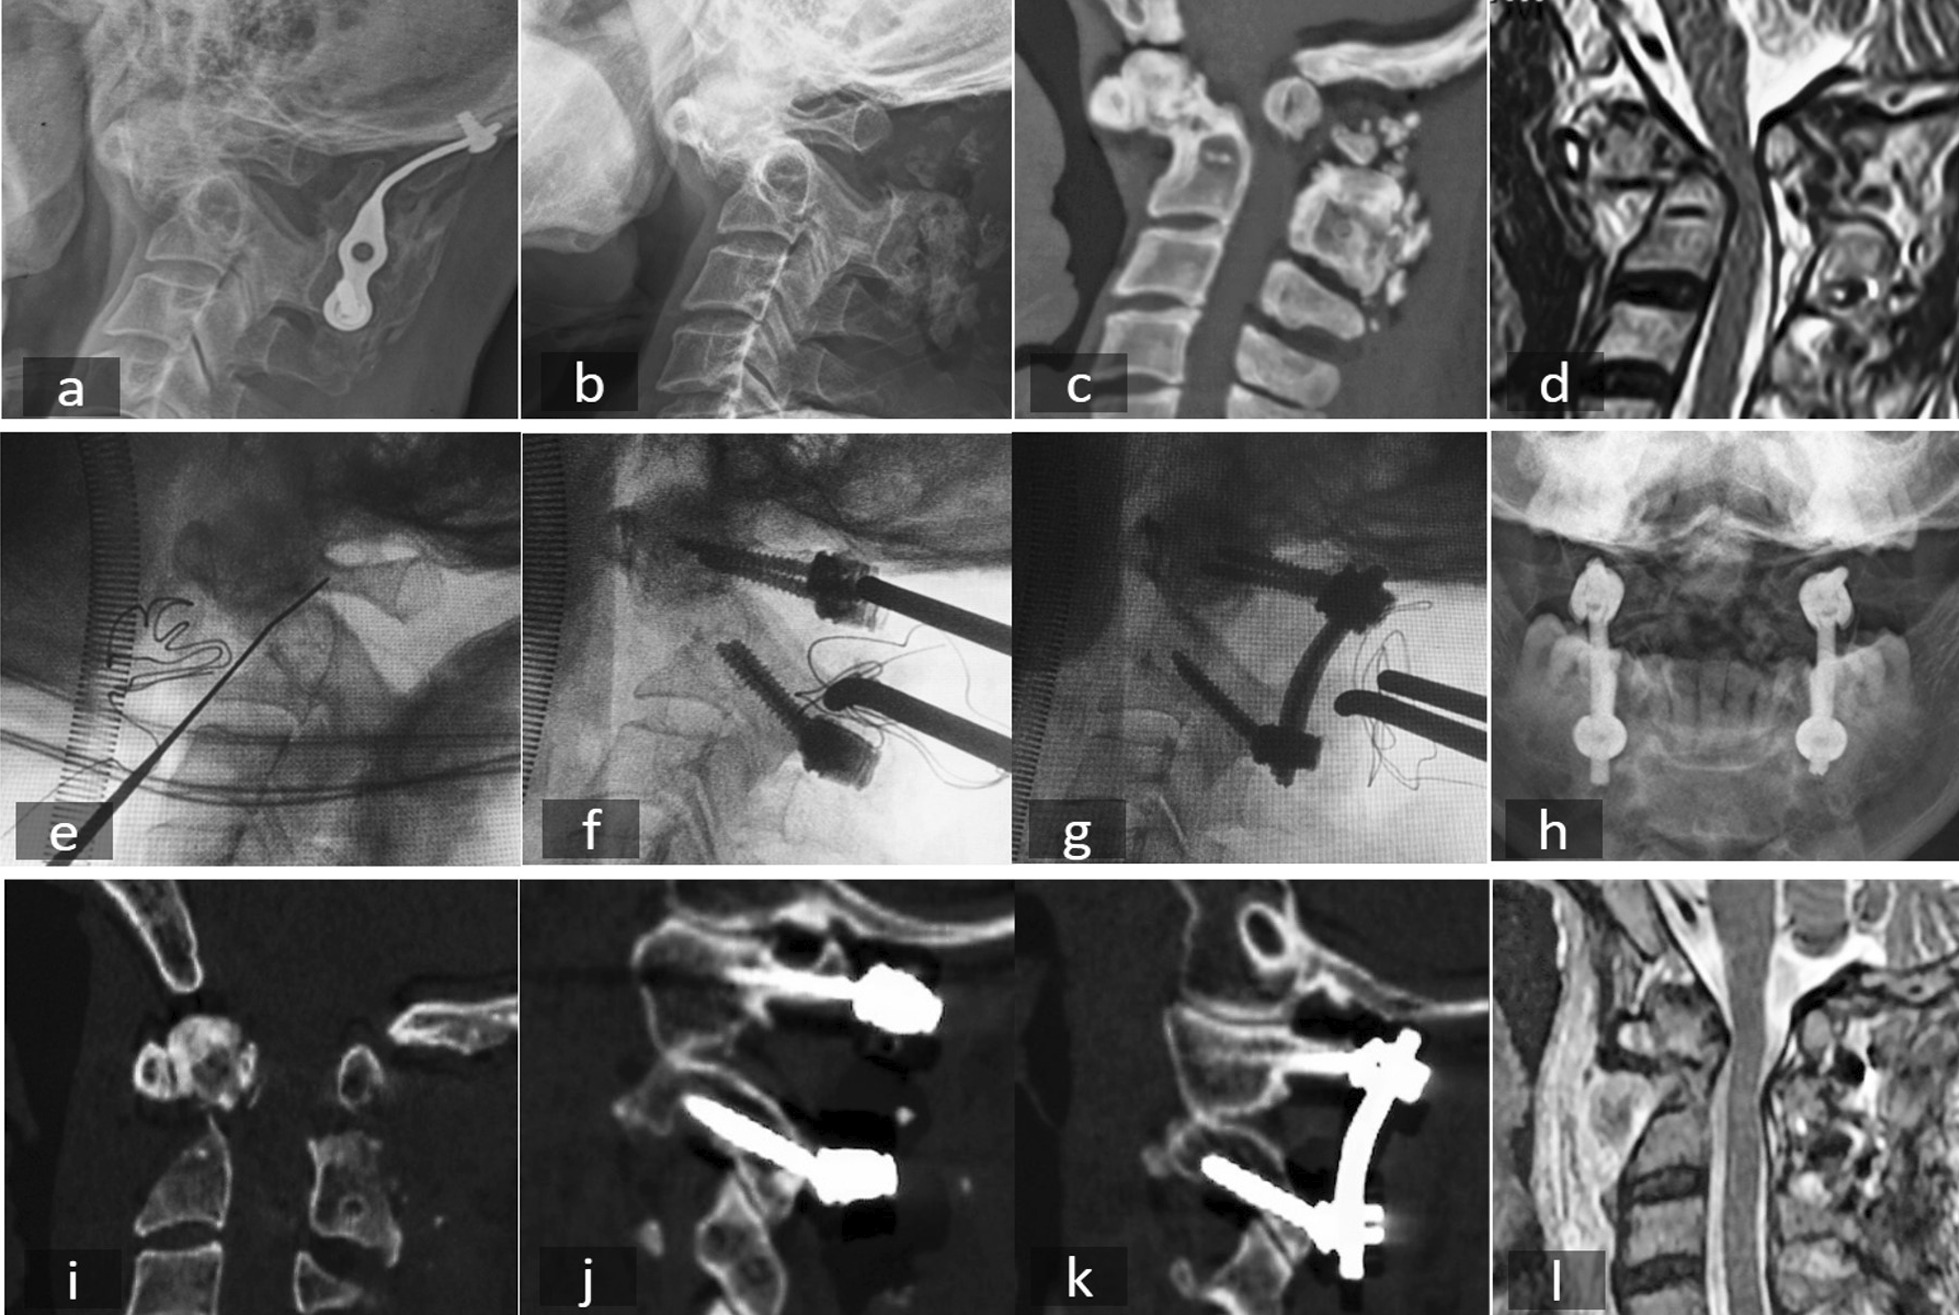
A 43-year-old man with fracture of C2 combined with atlantoaxial dislocation. a Preoperative X-ray showed C0-C2 bone graft and fusion was performed due to fracture malunion and dislocation of C1-C2 19 years ago. b, c Preoperative X-ray and CT scan showed malunion of C2 fracture. d Preoperative MRI showed atlantoaxial dislocation and spinal cord compression. e Intraoperative X-ray showed anterior release and osteotomy of odontoid. f Intraoperative X-ray showed the position of C1 PS combined with modified C2 isthmus screw land g Reduction. h Postoperative anterior–posterior X-ray, and i–k postoperative CT scan imagines showed good reduction and position of modified C2 isthmus screw. l Postoperative MRI showed the decompression of spinal cord. *PS* pedicle screw
